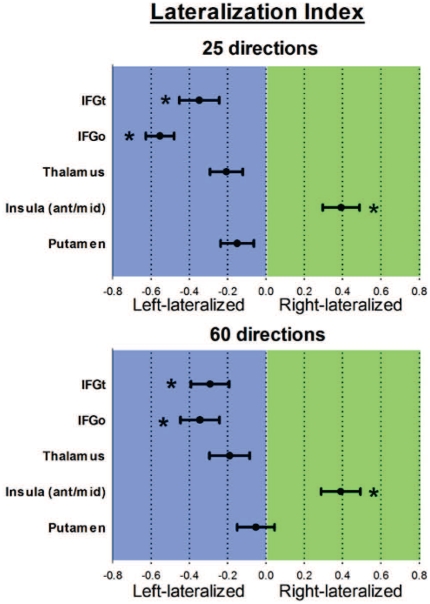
Lateralization index values for each target's connectivity with the TPJ. One sample t-tests revealed significant right lateralization of TPJ-insula connectivity as well as left lateralization of TPJ-IFGo and TPJ-IFGpt connectivity using both DWI acquisitions (*p*<0.05, Bonferroni-corrected). Abbreviations: IFGt = inferior frontal gyrus (pars triangularis), IFGo = inferior frontal gyrus (pars opercularis).
